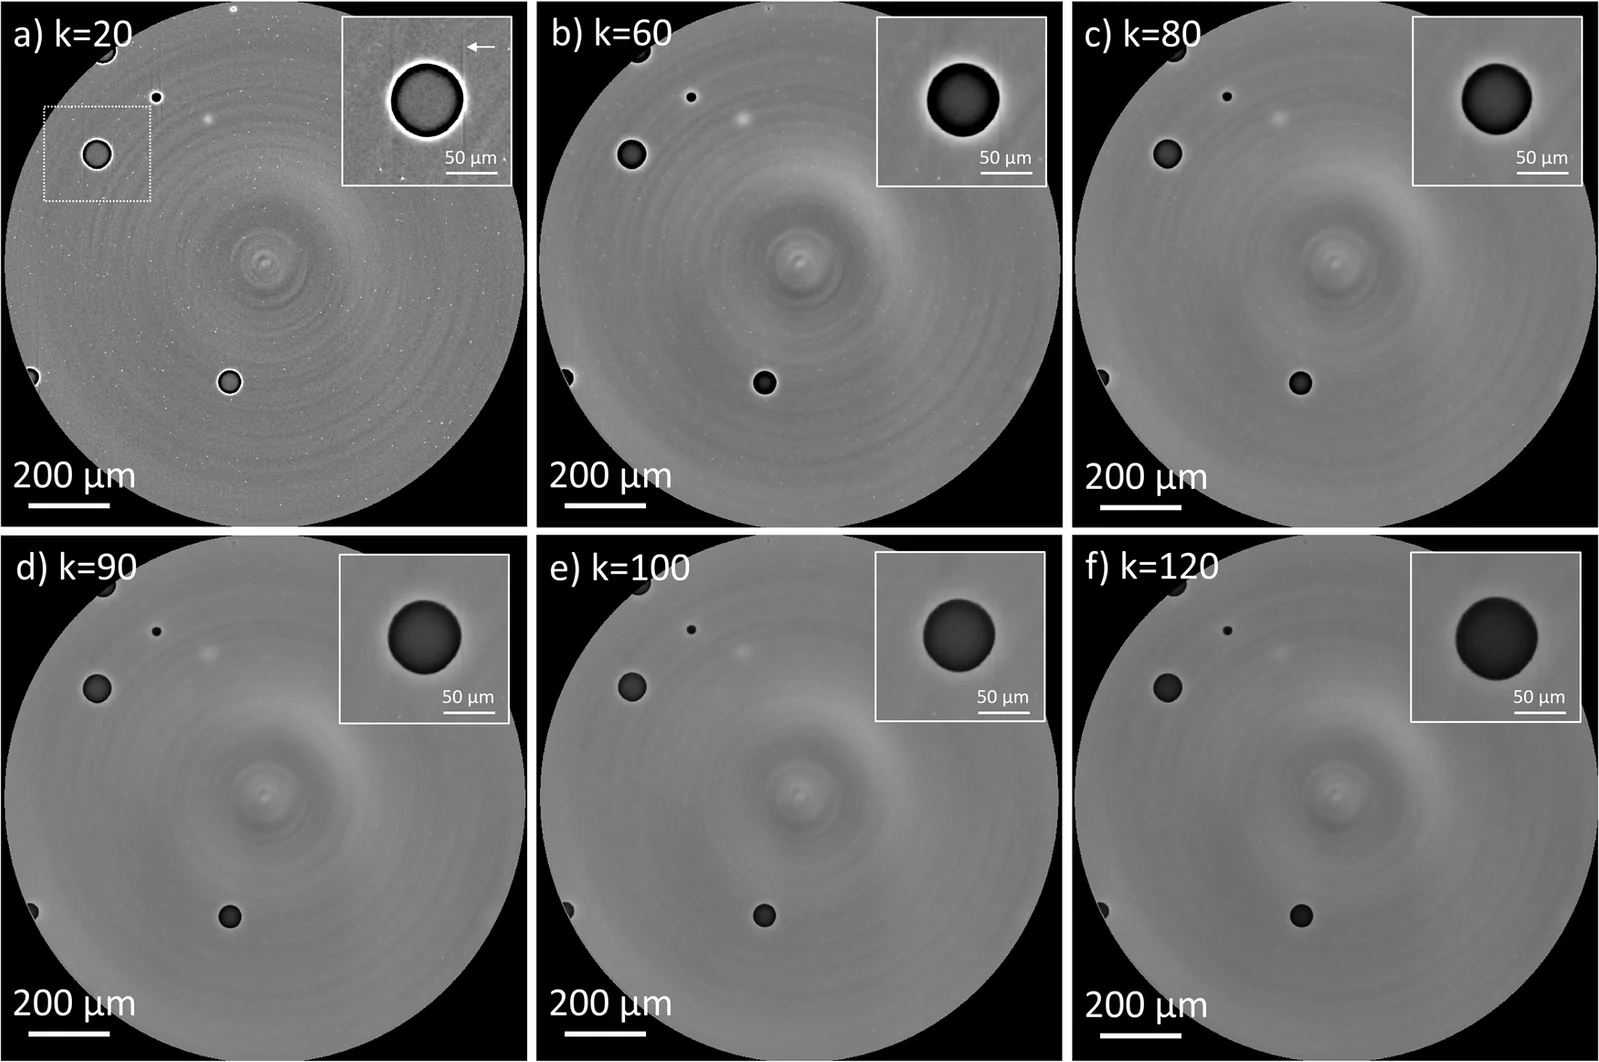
Depiction of the optimisation process for the Paganin filter kernel size, k. a–f show, for the ClearFlex50 sample, the central slice of each of the CT reconstructions at different values of k. The insets show the sphere highlighted in (a) at higher magnification. The value of *k* was chosen to minimise the phase fringes (see white arrow in the inset of (a)), around each microsphere whilst preserving image clarity.
